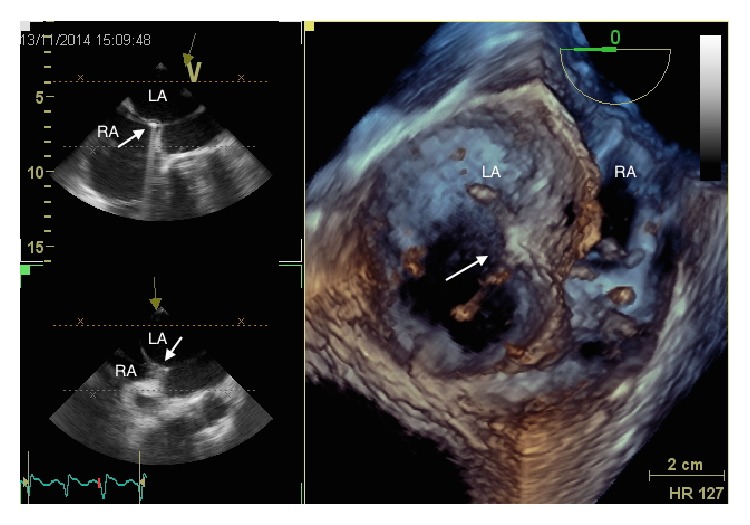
Real-time 3D echocardiography during transseptal puncture. The tip of the catheter (arrow) is passing from the right atrium (RA) to the left atrium (LA), through interatrial septum.
